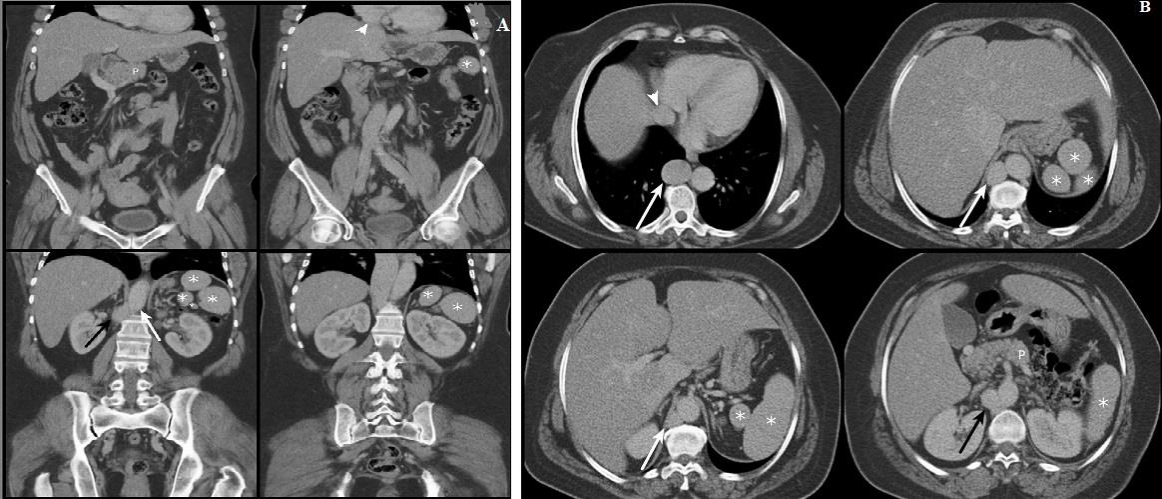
computed tomography scan with coronal (A) and axial (B) views showing the different anomalies found in the polysplenia syndrome in our patient
